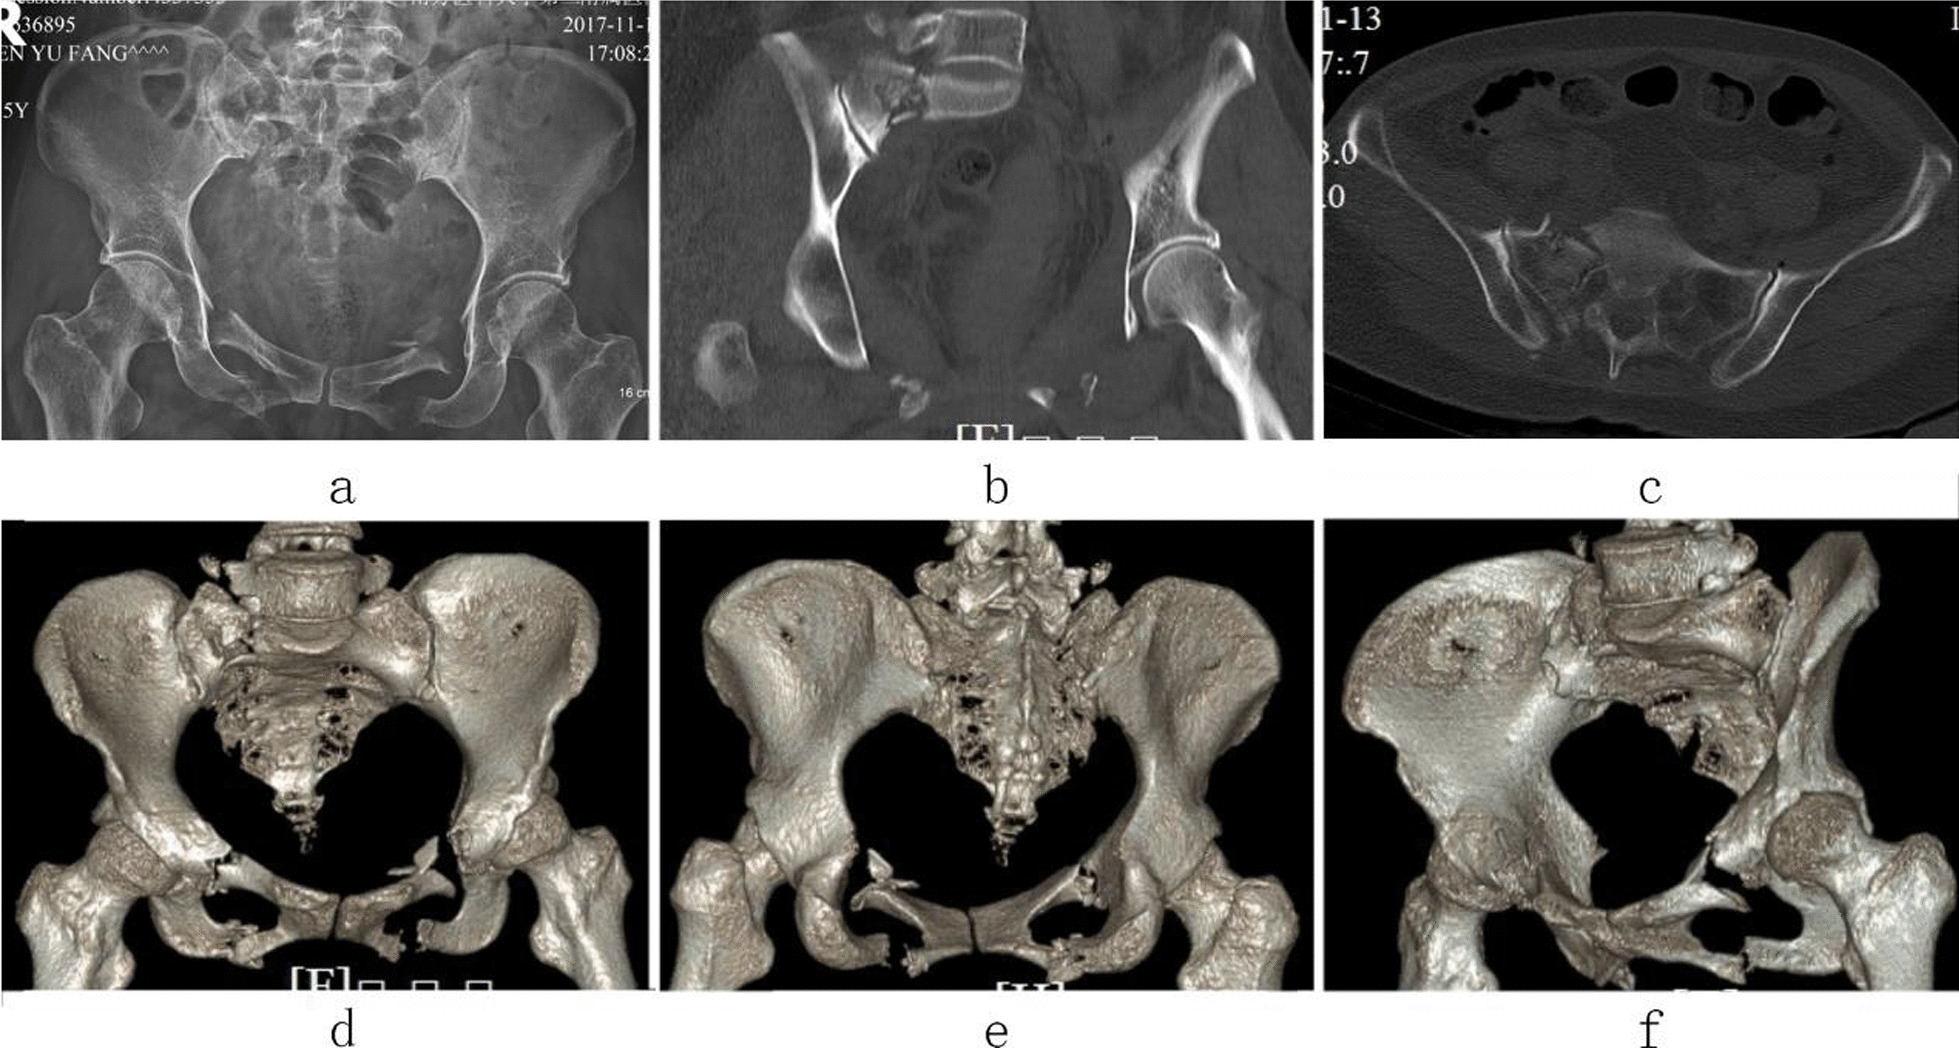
Preoperative images of case 2. (a Pelvic anteroposterior X-ray image, b CT sagittal image, c CT horizontal slice image, d–f CT 3D reconstruction images.)
